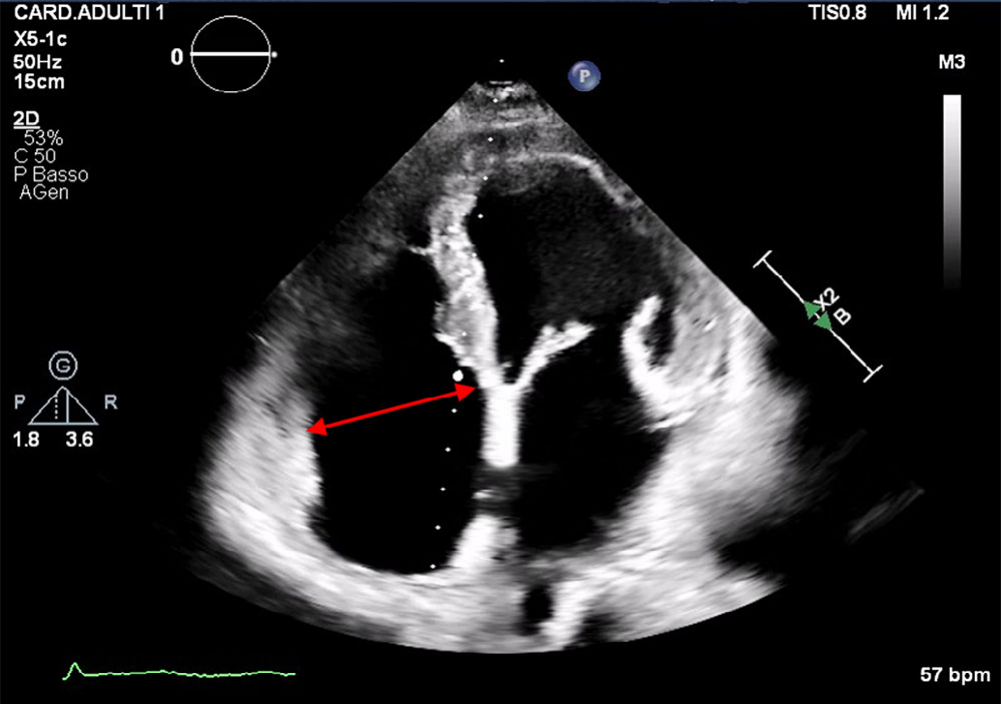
TTE four chambers view at tele‐diastole. Red two‐headed arrow pointing the measurement for the TV anulus. TTE indicates transthoracic echocardiography; TV, tricuspid valve.
